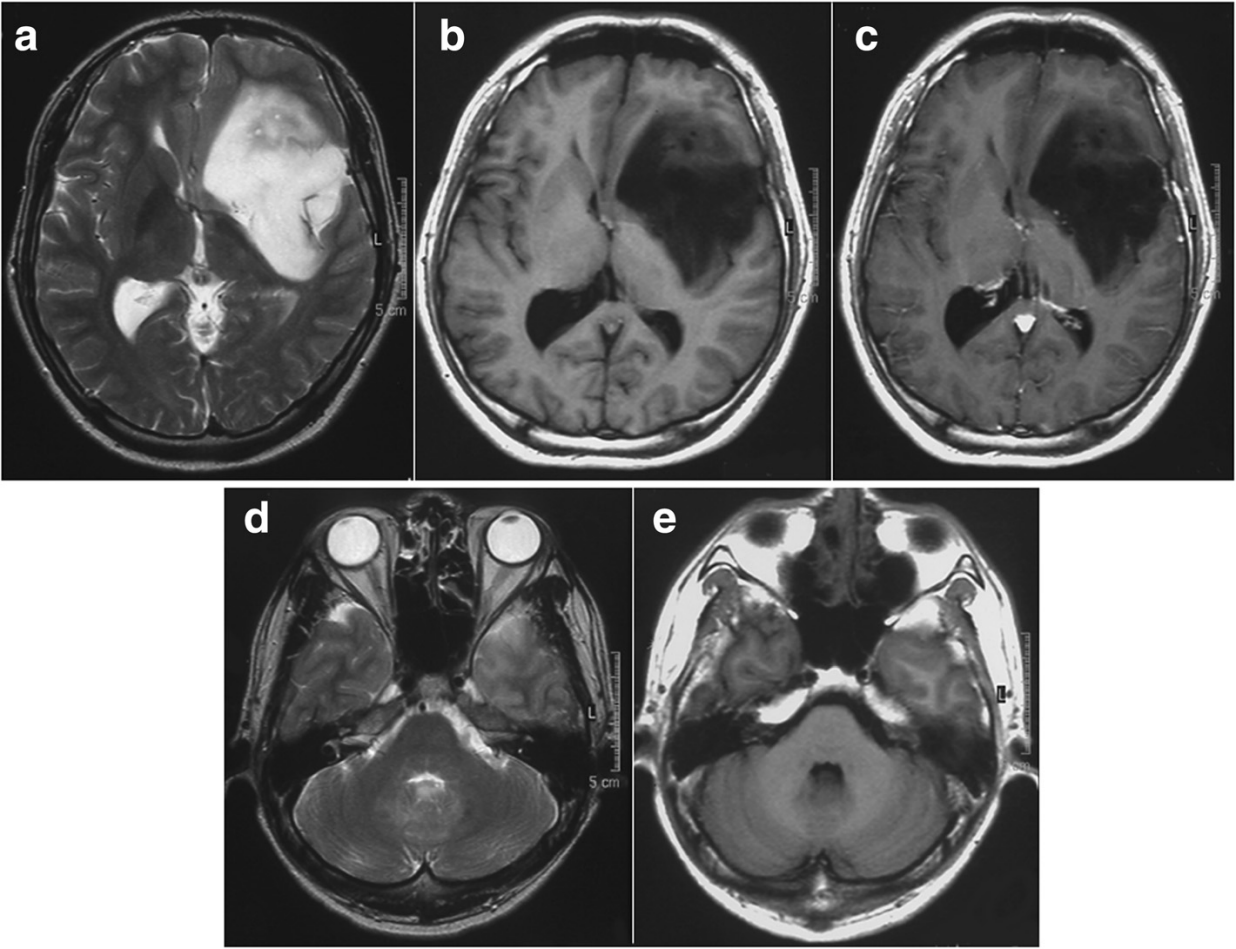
Preoperative axial T2-weighted (a), T1-weighted (b), and gadolinium (Gd) enhanced T1-weighted (c) magnetic resonance (MR) images showing a tumor mass in the left insula with a mild mass effect. The tumor was not enhanced on the administration of Gd. Axial T2-weighted (d) and Gd enhanced T1-weighted (e) MR images obtained at the same time showing no tumor in the cerebellum
